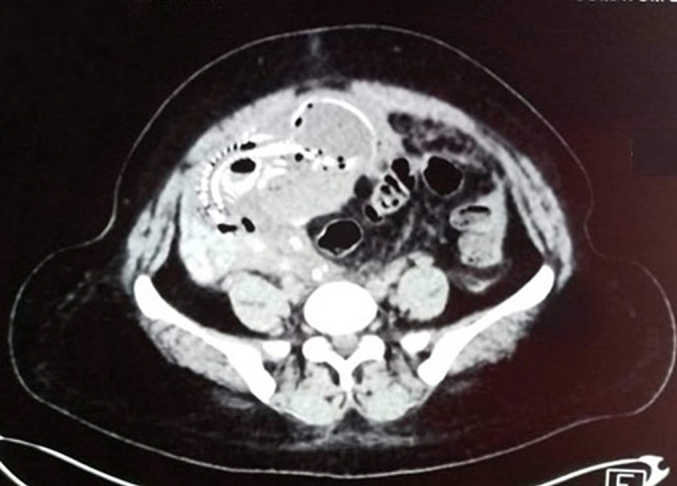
Identification of the fetal bone skeleton in the pelvic area - Captured by abdominal CT, this figure highlights the fetal bony structure located within the pelvic region, a key diagnostic feature supporting the identification of an advanced abdominal pregnancy.
